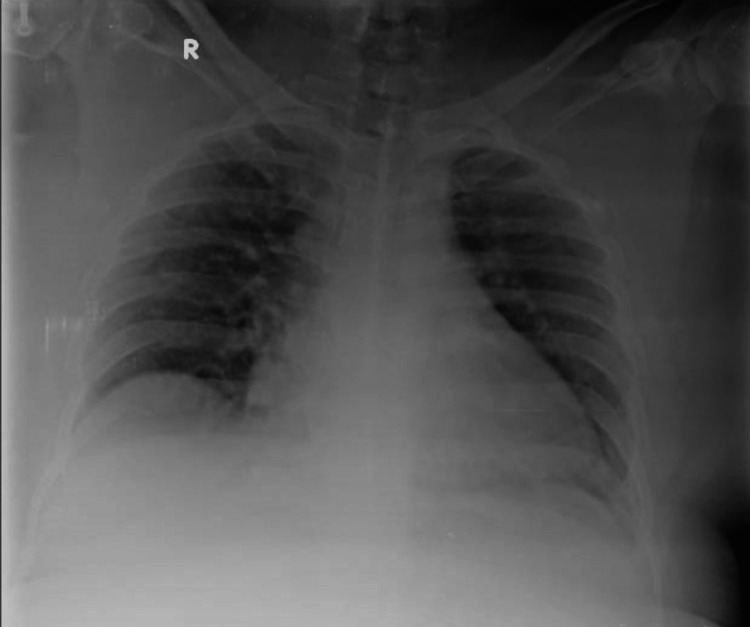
Admission chest X-ray revealing patchy airspace opacities.
